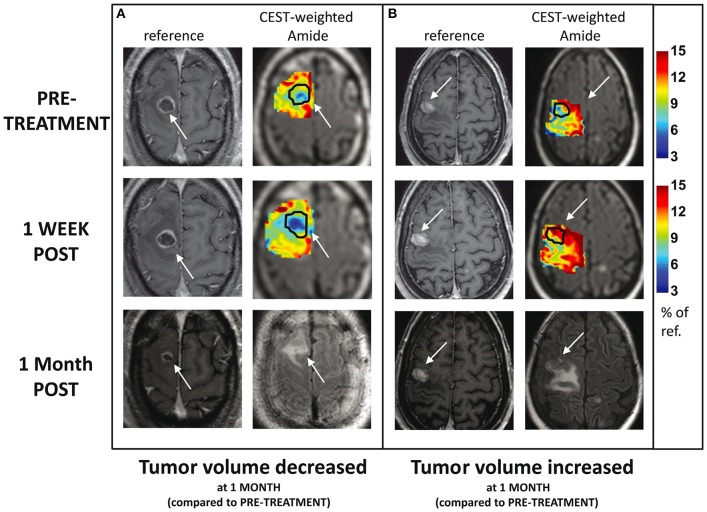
CEST amide MTR maps (tumor and surrounding tissue) for two patients with brain metastases treated with single-dose SRS, at baseline and 1 week after treatment: (A) the tumor volume decreased 1 month post-SRS and (B) tumor volume increased 1 month post-SRS. The maps are overlaid on T2w FLAIR images. The enhancing tumor region is indicated with arrows and outlined on CEST maps. For comparison, the corresponding slice from the post-Gd T1w MRI is also shown at all three scan time-points. Reproduced, with permission, from Desmond et al. (69).
